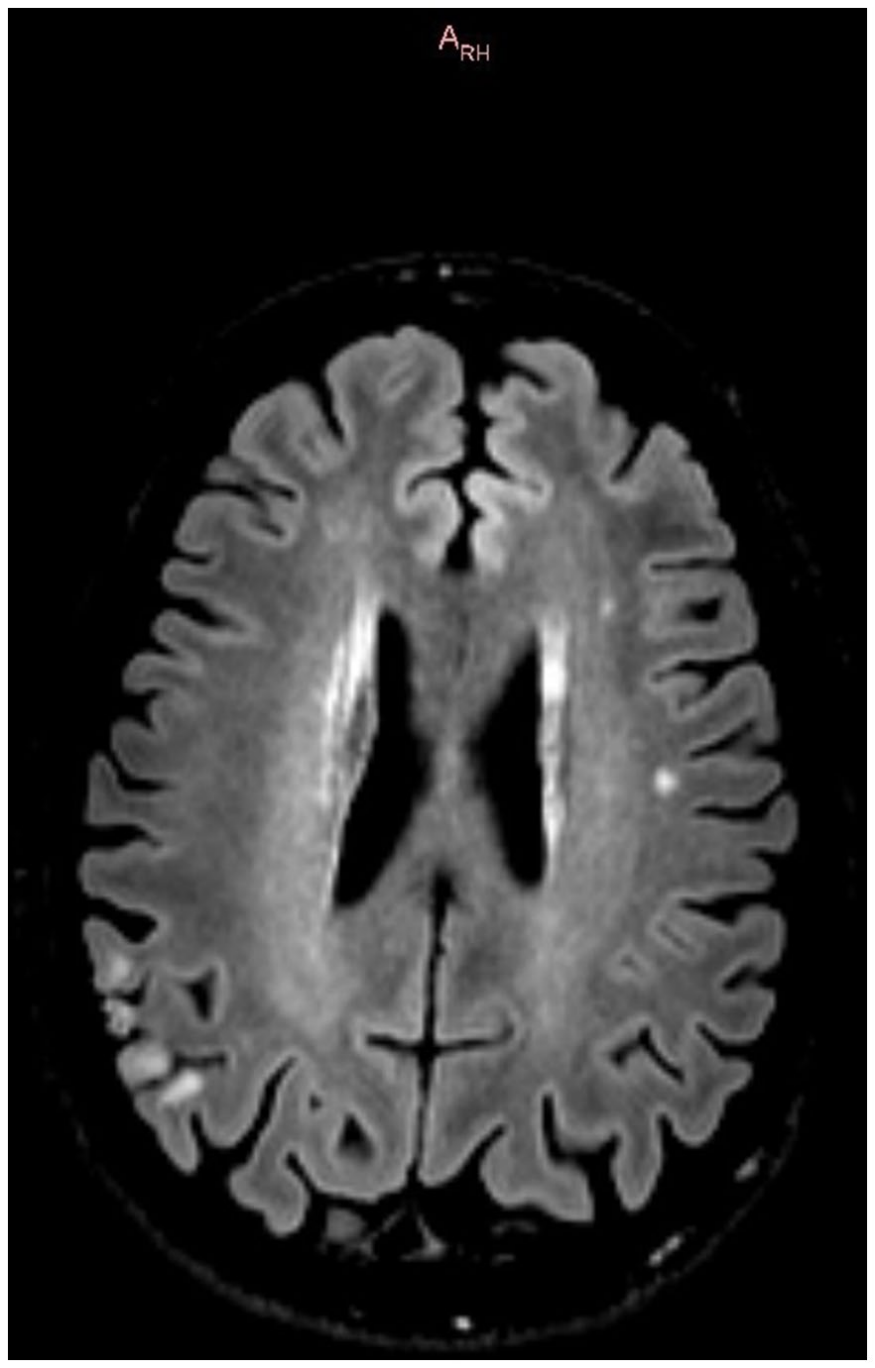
3T brain MRI displaying basal ganglia calcification and left temporal stroke, 15.11.2023.
